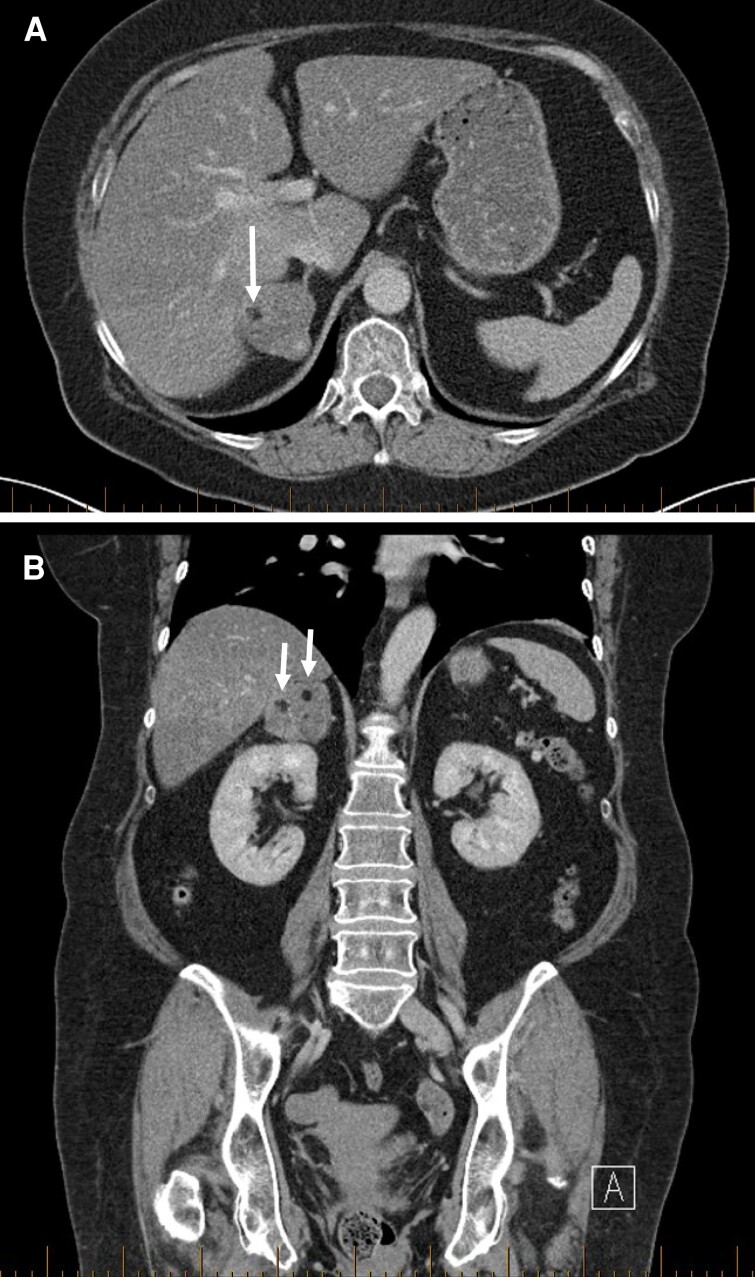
(A) Case 1, initial abdominal CT, axial portal venous phase. Focus of macroscopic fat indicated by arrow. (B) Case 1, initial abdominal CT, coronal portal venous phase. Two foci of macroscopic fat indicated by arrows.
